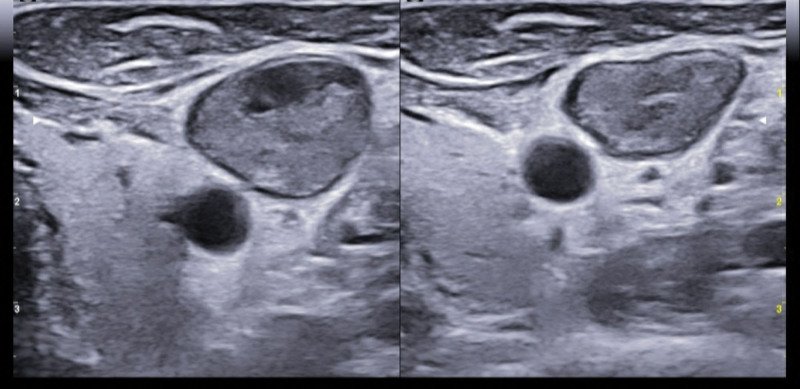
Ultrasonography baseline-images: short axis view of the left jugular vein (compression ultrasound CUS maneuver) and long axis view of the left jugular vein and subclavian vein, overall showing deep vein thrombosis.
